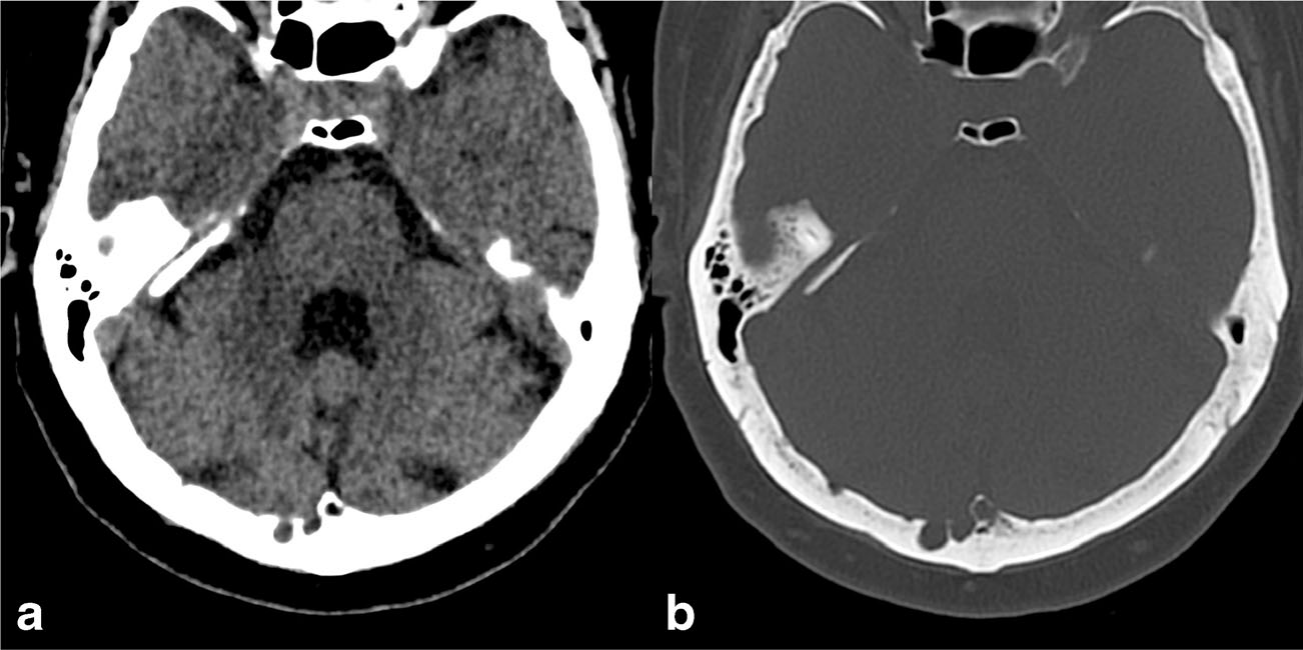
Arachnoid granulations (AGs). Axial computed tomography (CT) images with brain (a) and bone (b) windows showing two well-defined, round-shaped lesions with cerebrospinal fluid (CSF) density, protruding into the calvarium along the right transverse sinus
